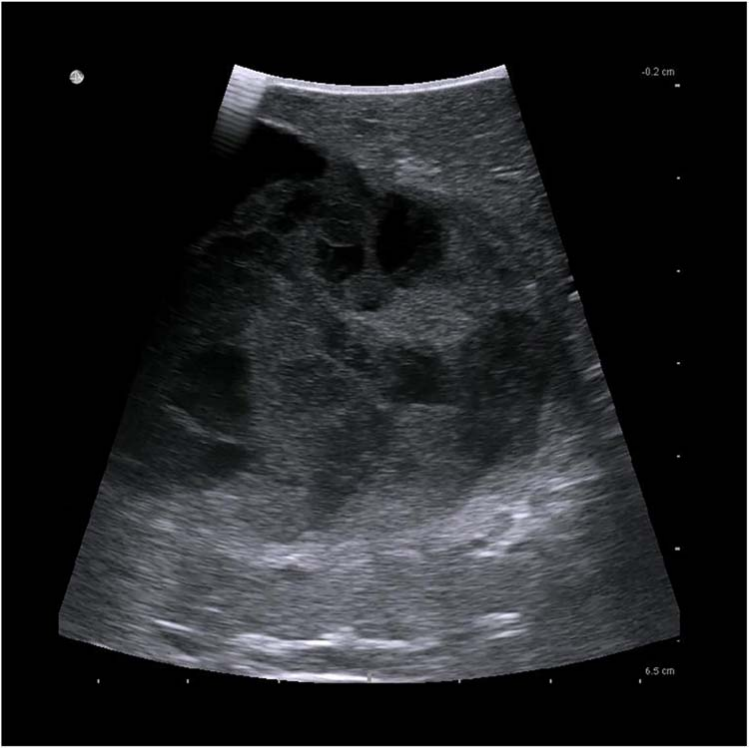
Intraoperative ultrasonographic localization of the liver cyst.
